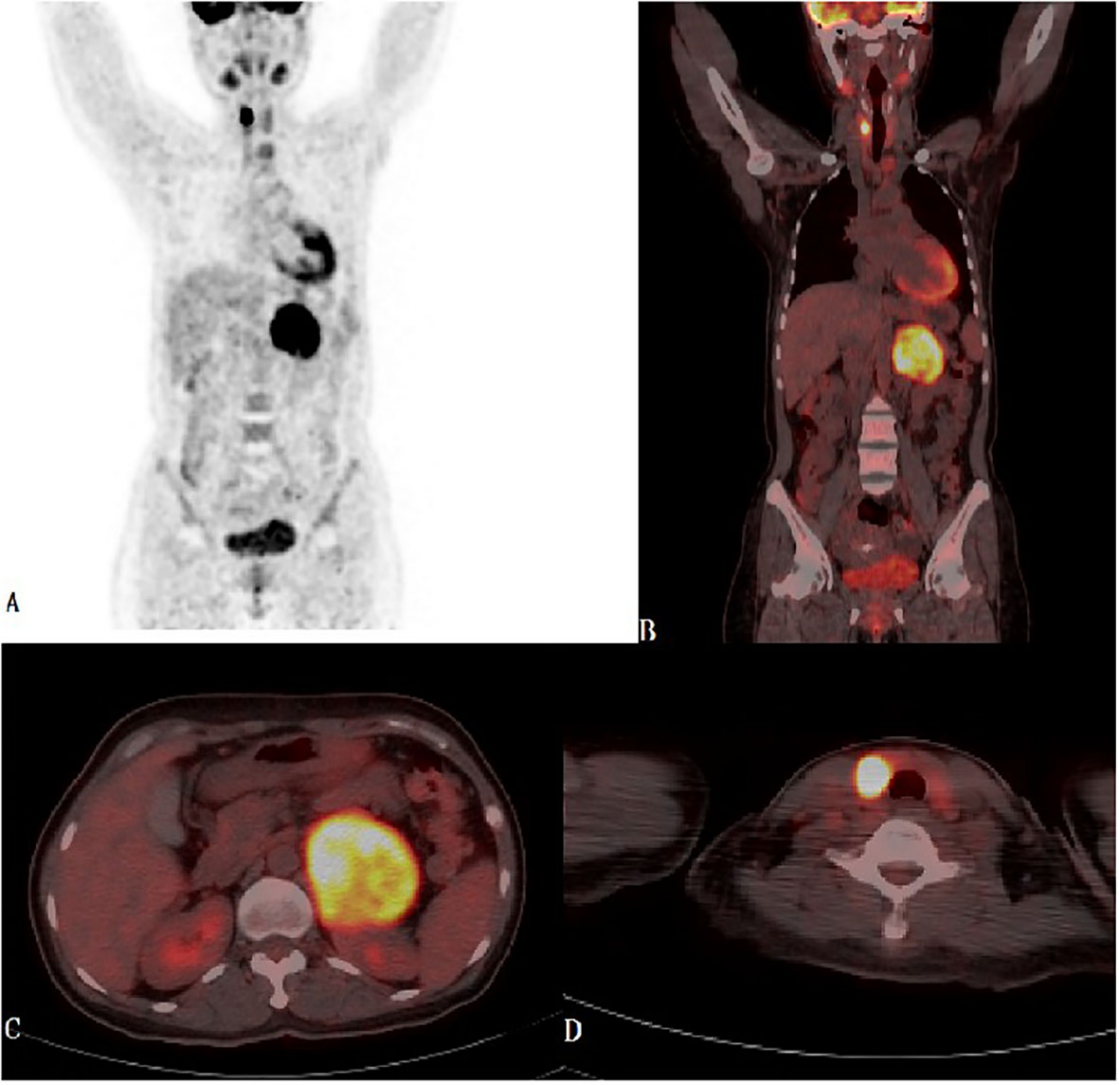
(A-D) Maximum Intensity Projection-MIP-PET and PET-CT fused images in coronal and transaxial projections showed left bulky retroperitoneal mass with heterogeneous intense FDG uptake and focal intense FDG uptake in the right thyroid lobe.
